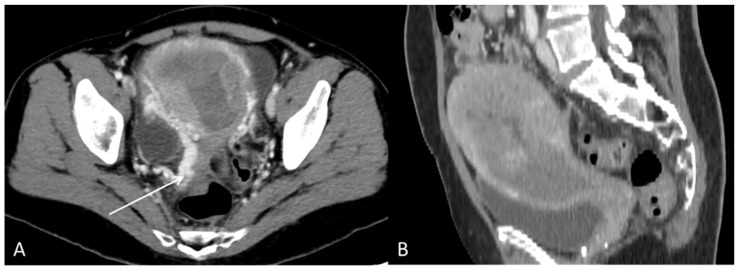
The CT with contrast enhancement performed after approximately two months of sorafenib treatment showed a morphological progression of the primary tumour in the uterus. During this time, the patient had also received radiation therapy (3Gyx10) of the uterus as palliative treatment due to heavy vaginal bleeding. (A) Axial CT and (B) sagittal CT showed tumour masses in the uterine cavity with patchy hypodensities consistent with necrosis. In the tumour periphery, contrast-enhanced varicose veins were visible, consistent with pelvic congestion syndrome ((A), arrow).
